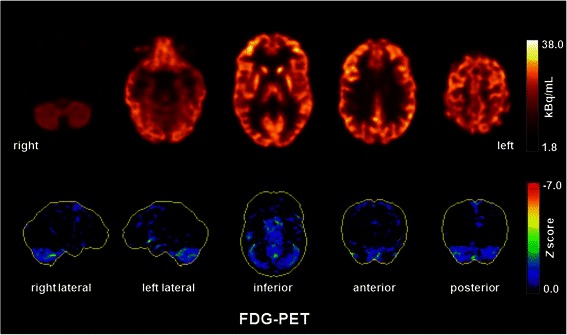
[^18^F]fluorodeoxyglucose positron emission tomography (FDG-PET) showed a mild, borderline significant cerebellar hypometabolism. Otherwise, the PET was unremarkable. No mesiotemporal hypermetabolism as typically seen in limbic encephalitis was observed. FDG-PET was performed at the Department of Nuclear Medicine of the University Hospital Freiburg after injection of 320 MBq FDG (Gemini True Flight, Philips Electronics, The Netherlands). Upper and lower row images show transaxial FDG-PET images and 3D surface projections of regions with decreased FDG uptake (colour-coded *Z*-score, compared to age-matched healthy controls), respectively.
